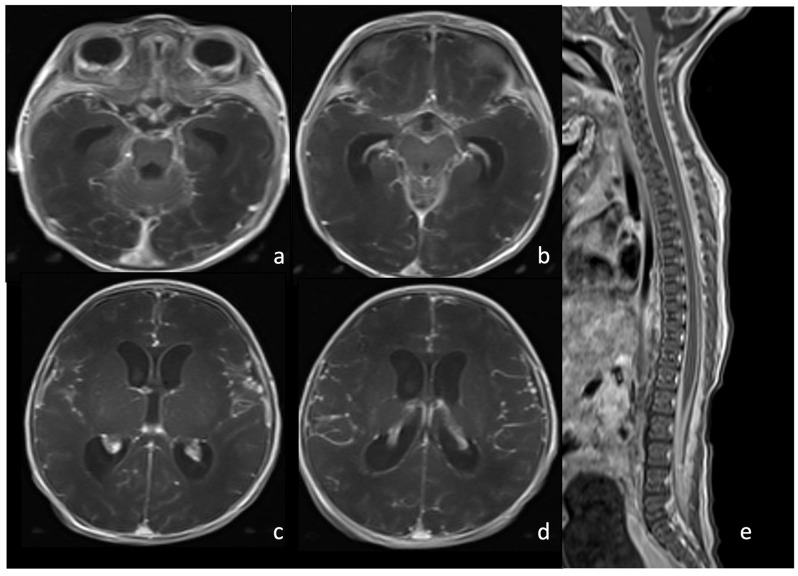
Newborn affected by *Escherichia Coli* meningitis and undergoing brain and spine MRI. Axial post-contrast T1WI of the brain (a–d) and sagittal post-contrast T1WI (e) of the spine show intense and diffuse pachymeningeal and leptomeningeal enhancement.
